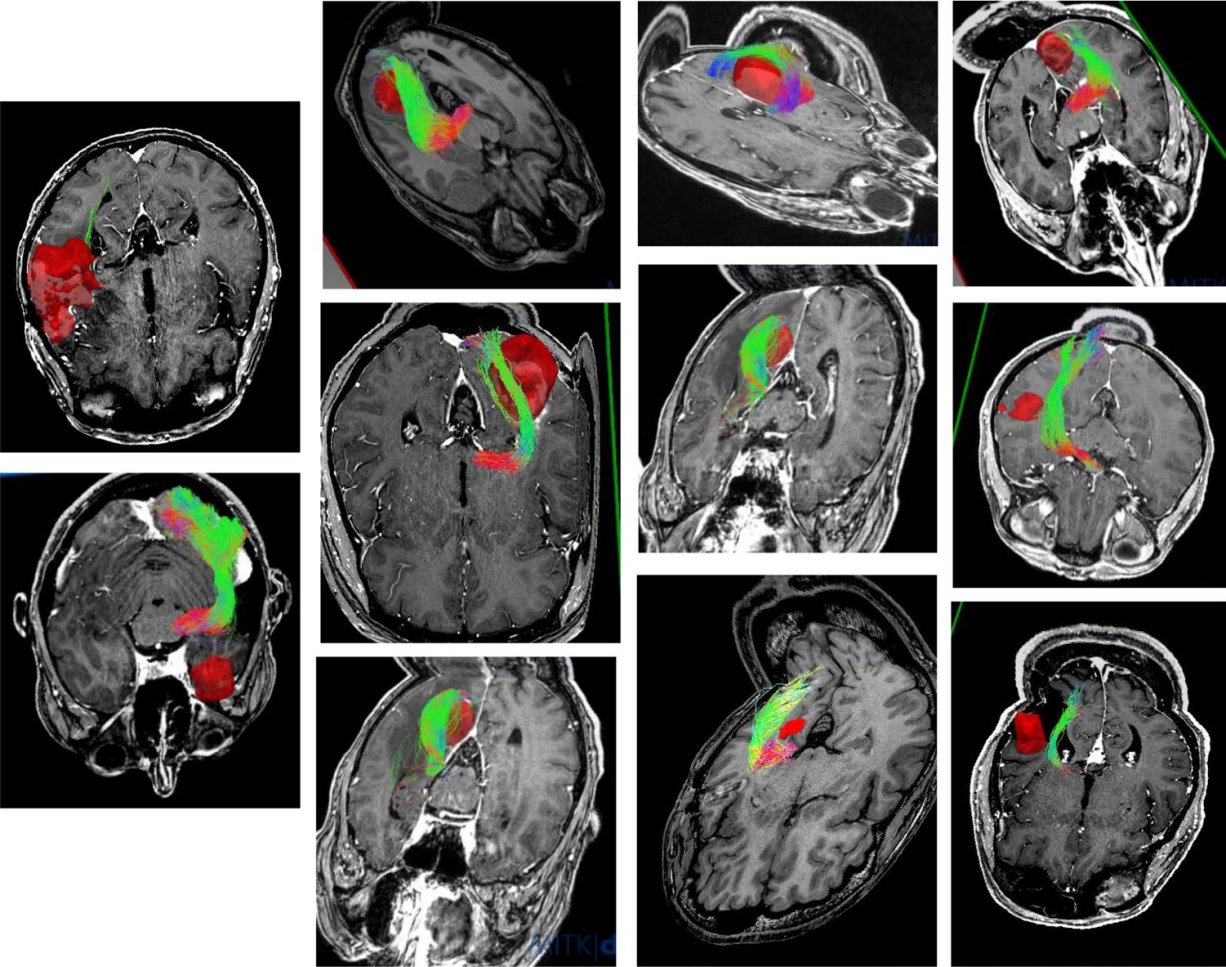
Overview of OR FT results. Tumors displayed in red. MRI T1-weighted, 3D view, cropped. All Rater 1, QBI-FT. (OR, optic radiation; QBI, Q-ball Imaging; FT, fiber tractography)
